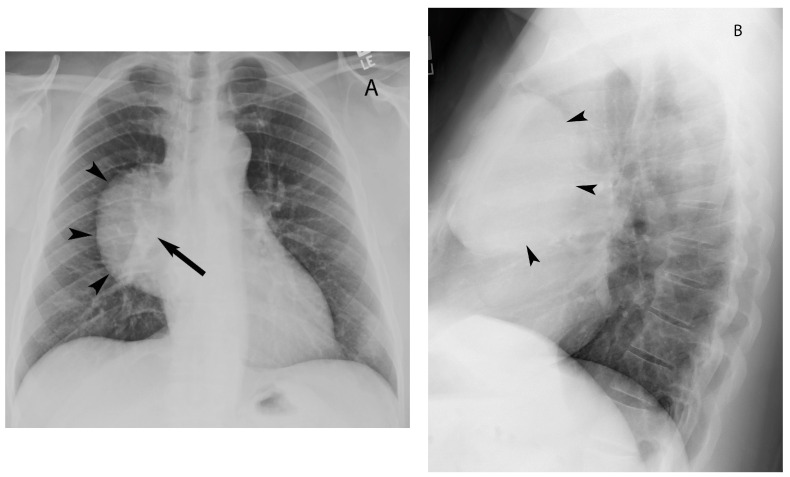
Hilum overlay sign. Chest radiograph posteroanterior view (A) shows well-marginated mass over right hilum (arrowheads). Hilar vessels (arrow) are seen through the mass, suggesting the mass is either anterior or posterior to the hilum. Chest radiograph lateral view (B) confirms the anterior location of the mass (arrowheads).
